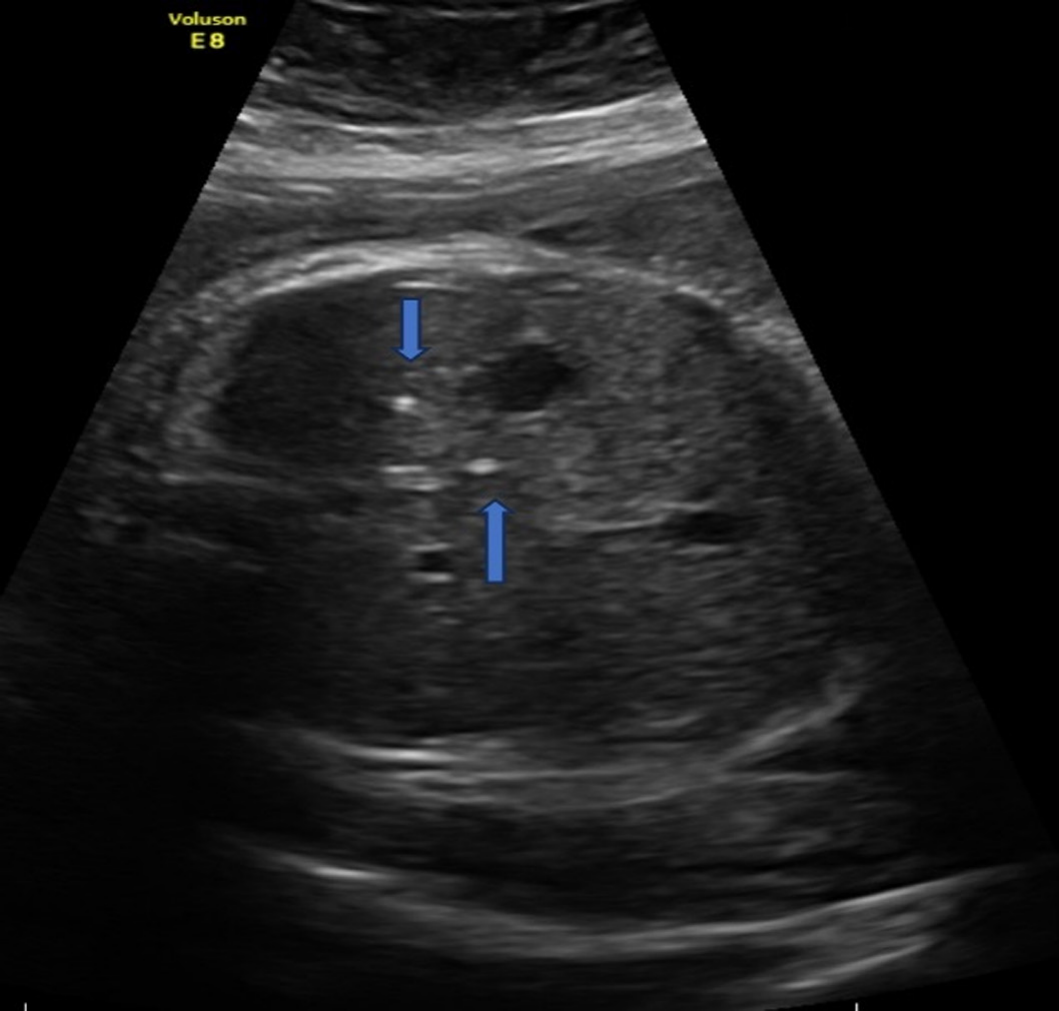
Ultrasound axial image of the fetal abdomen showing liver calcifications (Blue arrows).
